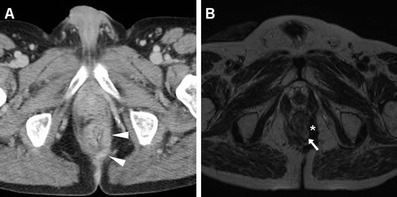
A 46-year-old HIV-infected man with biopsy-proven SCAC. Initially, MDCT (a) shows left-sided thickening with involvement of the external sphincter (*arrowheads*). After chemo-radiotherapy, lesion regression is observed on MRI with appearance of T2-hypointense fibrosis (*) and hyperintense nodule (*arrow*) consistent with residual tumour
